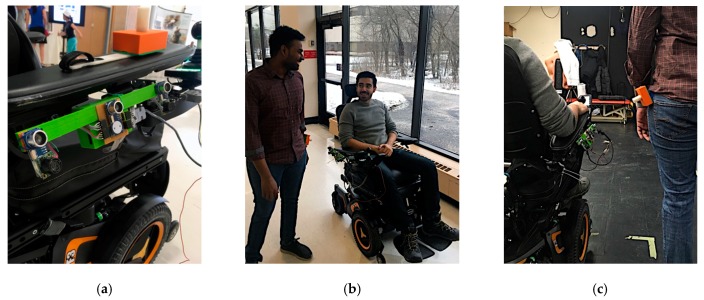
(a) Ultrasonic tethering system prototype mounted on a Permobil F5 corpus powered wheelchair, with AP beacon laying on the armrest, (b) Ultrasonic tethering system for social following, and (c) Ultrasonic tethering beacon placement on the accompanying person.
